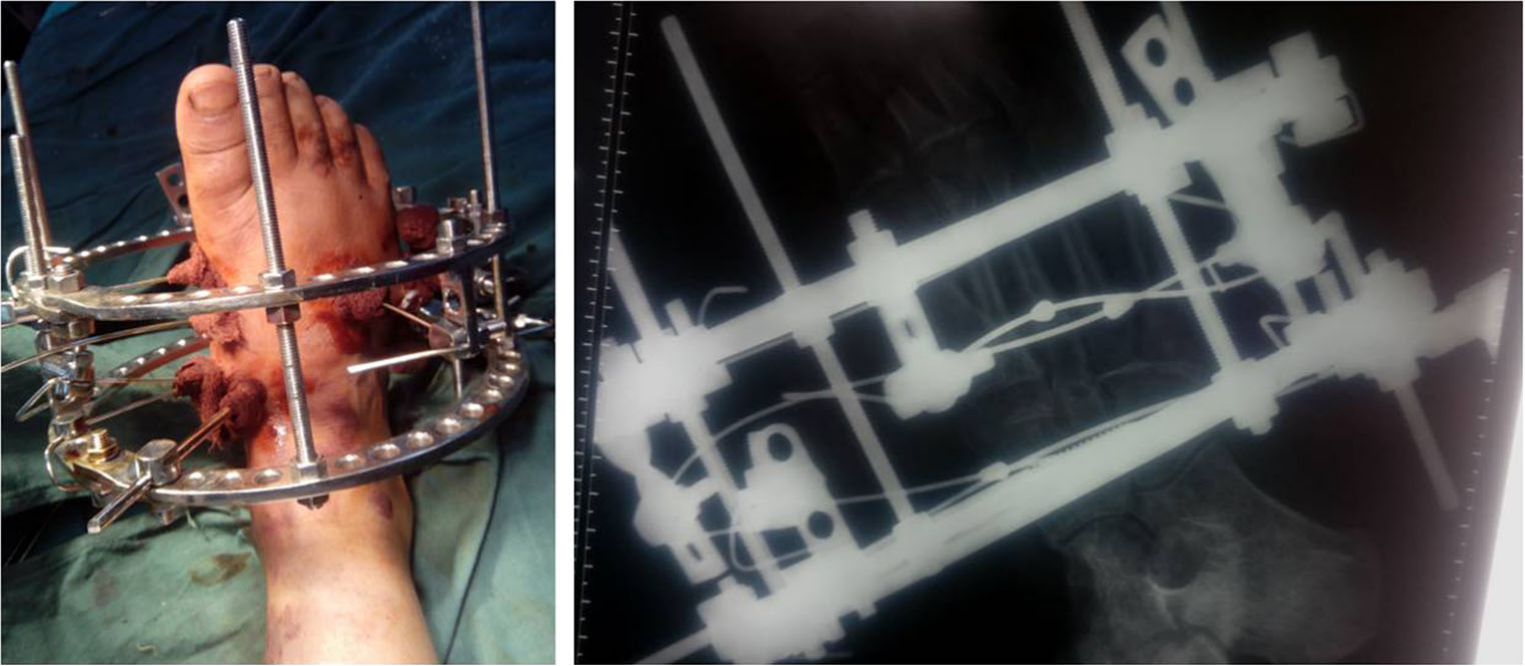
Clinical picture and X-ray on Ilizarov ring fixator
